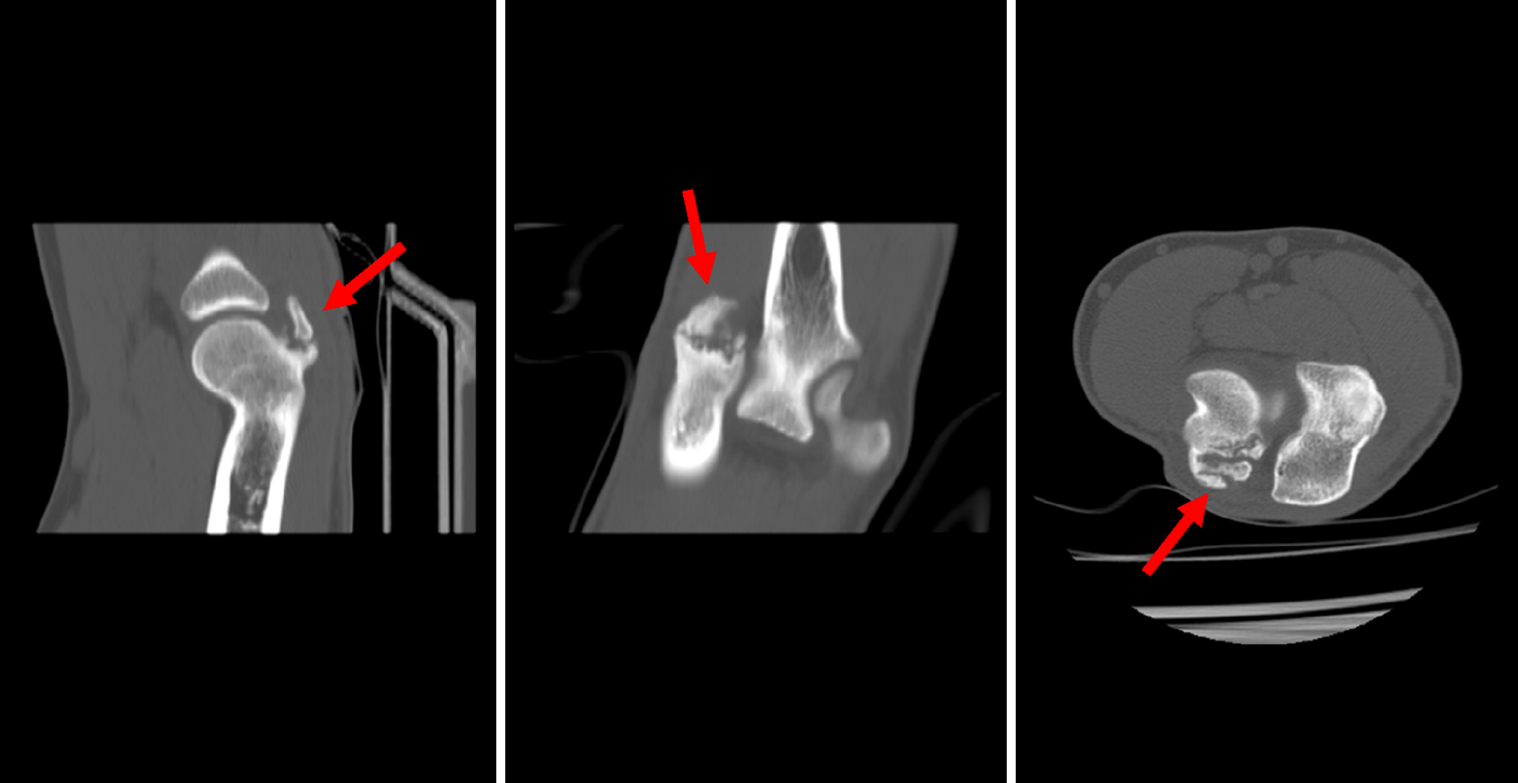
CT of the right elbow of patient no. 7 at first presentation (the arrow marks the ossification)
